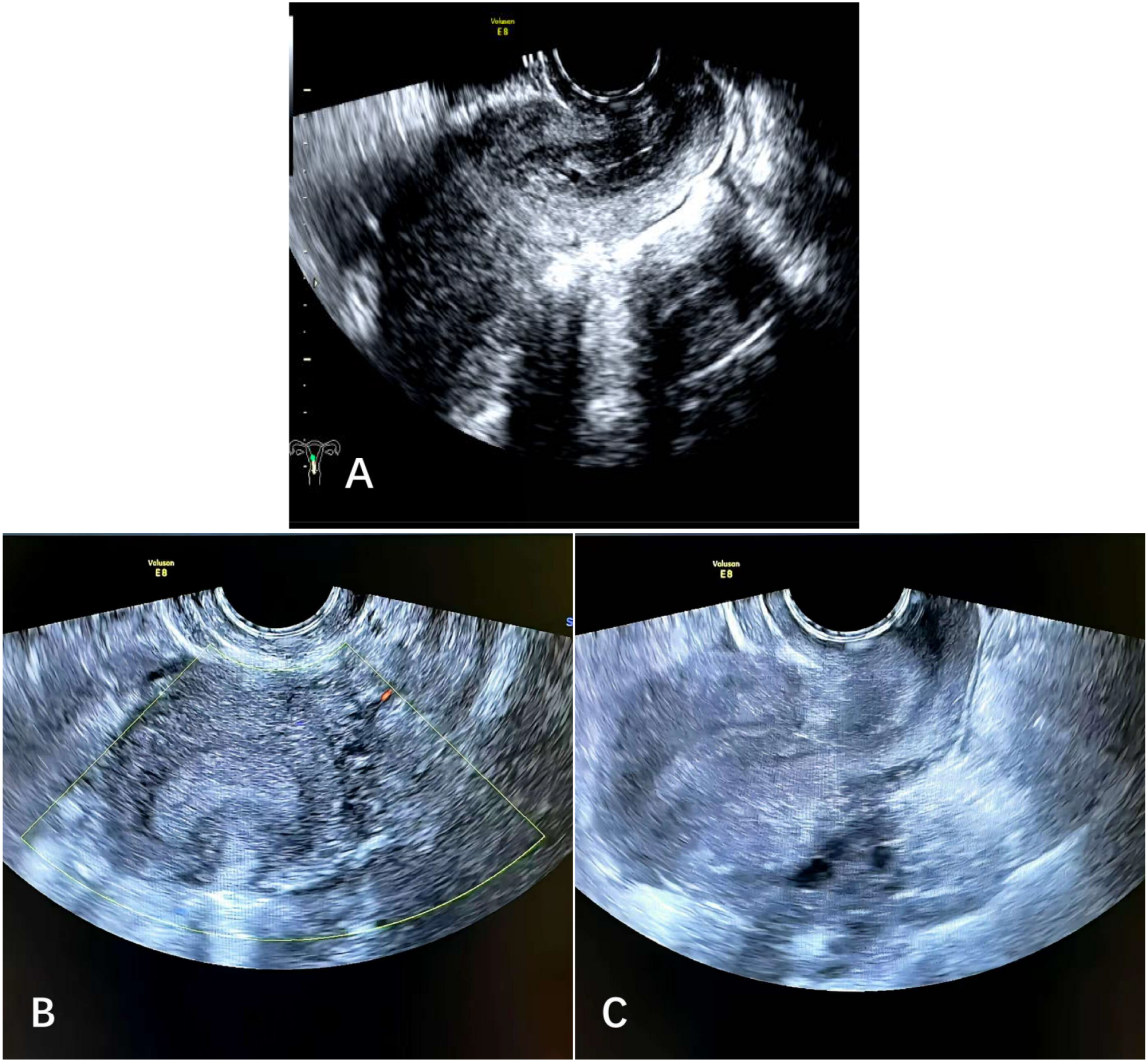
Transvaginal B-ultrasound re-examination one month after surgery showed no obvious abnormalities in the uterus and bilateral adnexa (A). Transvaginal B-ultrasound re-examination five months after surgery showed no obvious abnormalities in the uterus and bilateral adnexa (B, C).
